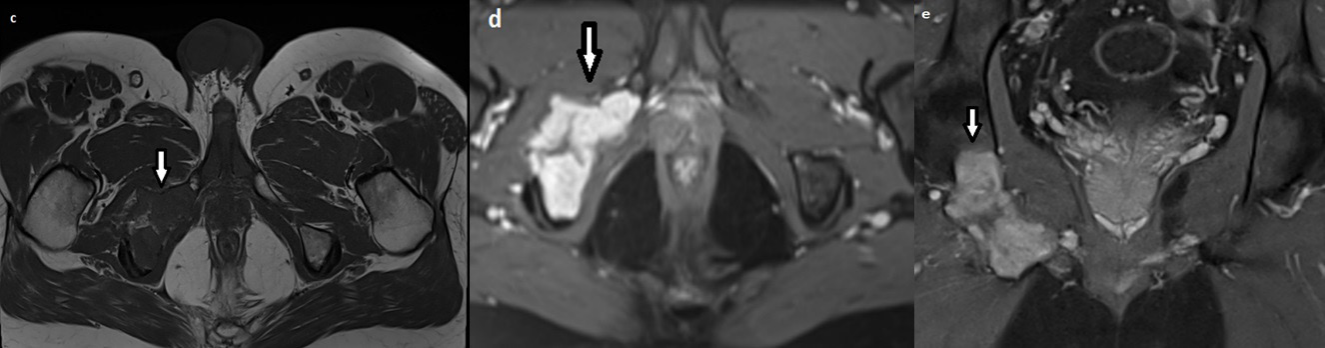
Pelvis MRI on axial T1 WS (c), axial (d) and coronal (e) T1 FS with gadolinium injection showing a lesion on the right ischio pubic ramus isointense on T1, enhanced after gadolinium injection with cortical destruction and soft tissue extension (arrow).
